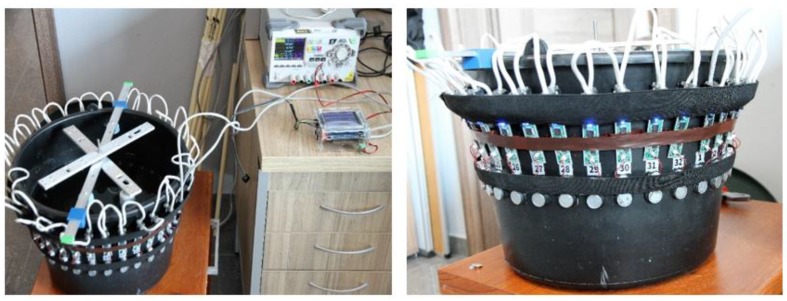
The developed ultrasonic system. A ring of 32 piezoelectric transducers mounted to the black bucket.
